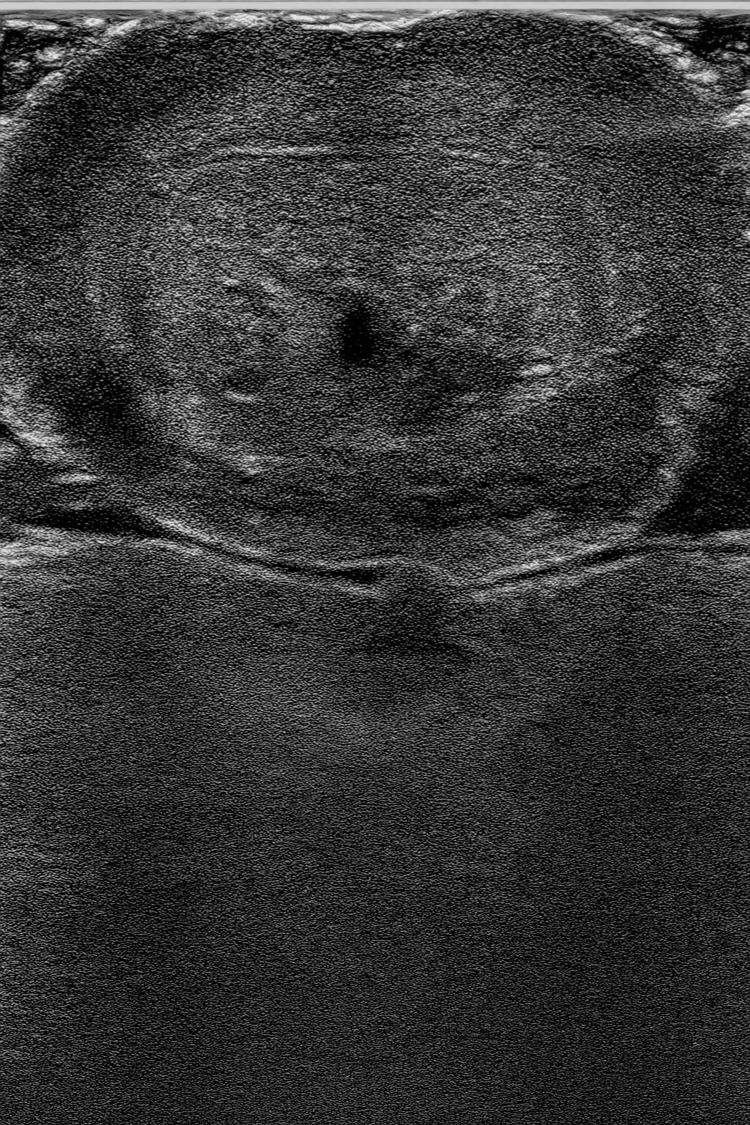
Axial section of the penis ultrasound showing edema of adjacent cellular tissue.
